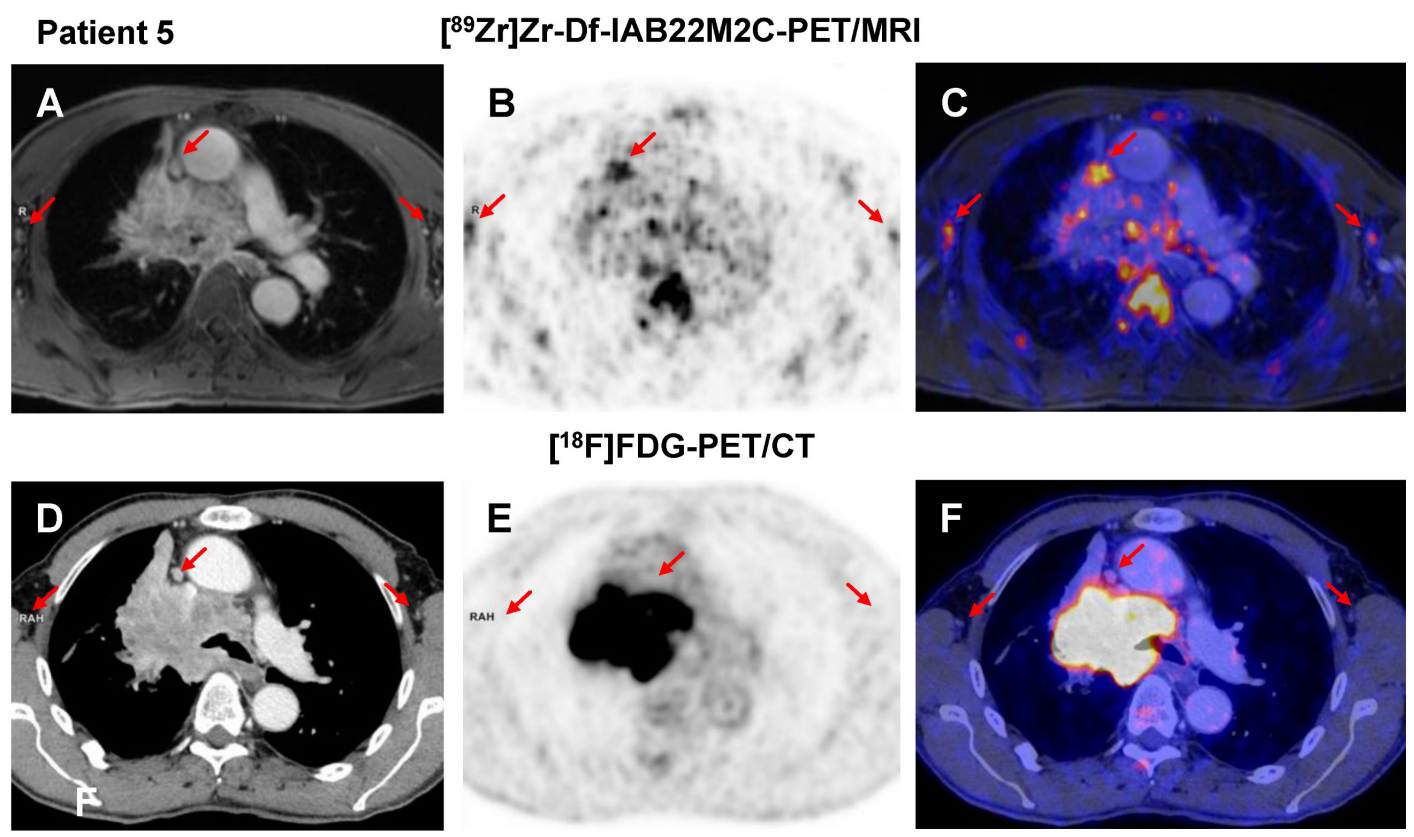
A-C: Patient 5 presented with moderate [^89^Zr]Zr-Df-IAB22M2C uptake in several mediastinal lymph nodes next to the primary tumor independent of any previous cancer treatment. D-F: In the previous [^18^F]FDG PET/CT scan, the mediastinal lymph nodes of Patient 5 were not considered suspicious for metastasis.
